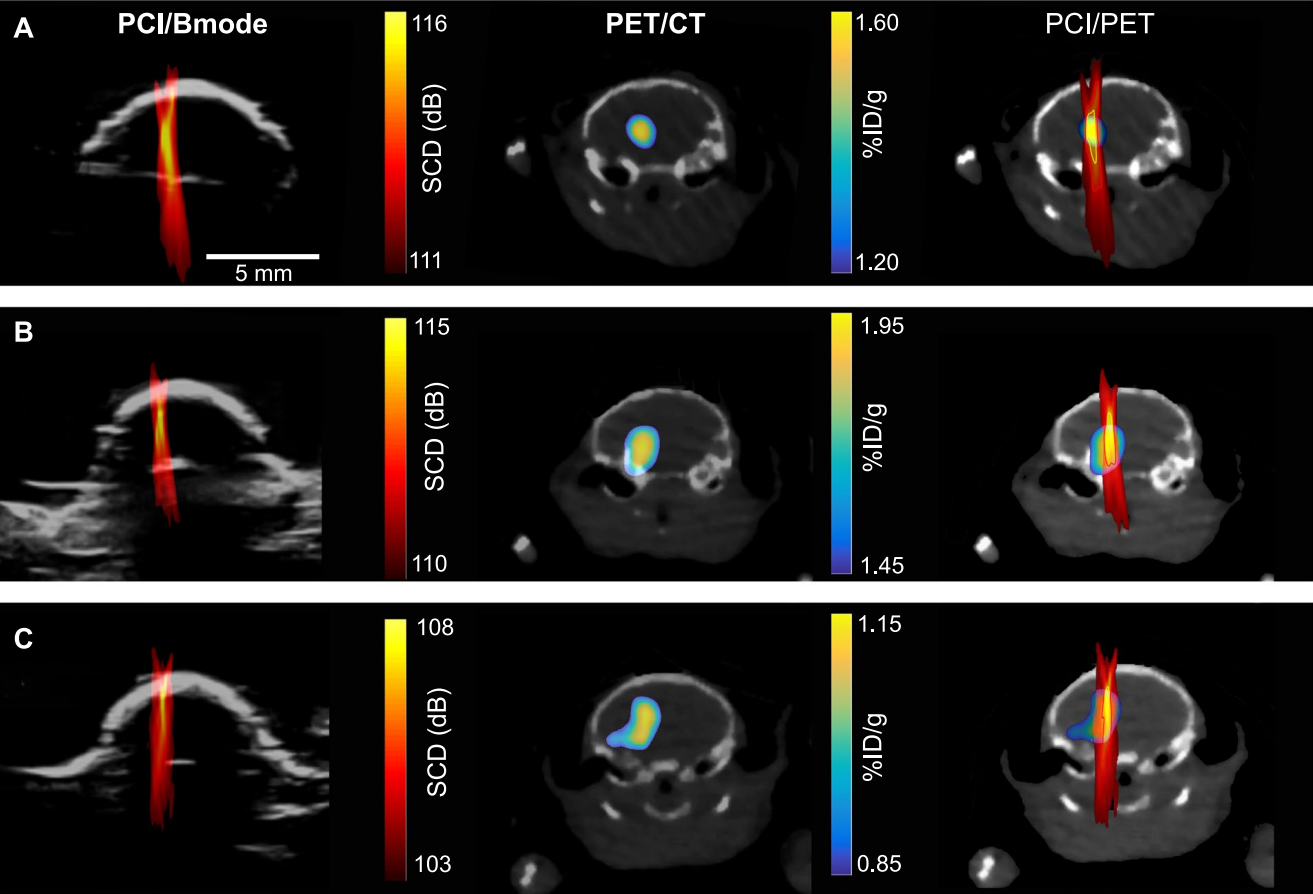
PCI/B-mode images (left column), PET/CT images (middle column), and PCI and PET overlaid images (right column) for three representative cases shown in A, B, and C, respectively.
